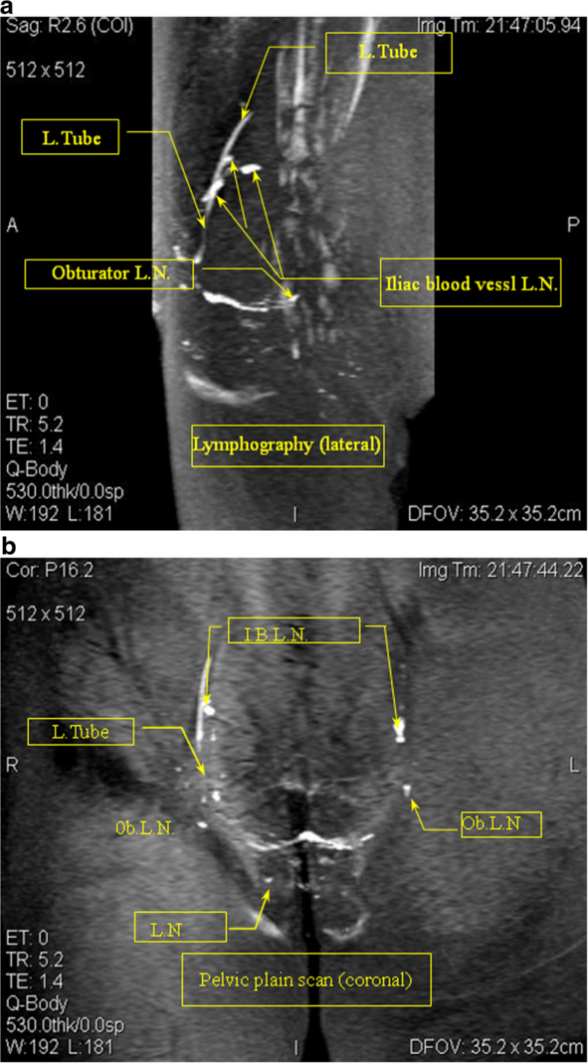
1–1: MR lymphography (sagittal view) Case 1: A 54-year-old cervical cancer patient. The MRI scan of the pelvic lymphatic system before lymphography showed no obvious lymph node metastases. The lymphography lateral map shows an irregular shape of the obturator lymph nodes (Ob. L.N.), and the iliac blood vessel lymph nodes (I.B. L.N.) show filling defects. The lymphatic shape was not continuous. MR lymphography in this patient was acquired using a slow subcutaneous injection of 15 ml Gadodiamide and 4 ml 2% lidocaine in the labia majora pudendi followed by a 30 second massage of the injection site. MRI shows the lymph nodes (L.N.) and lymphatic vessels (L.Tube). 1–2: Lymphography (coronal view) Case 1. The contrast with lymphatic (coronal view) irregular shape of the bilateral iliac blood lymph nodes (I.B.L.N.) and the right obturator lymph nodes (Ob.L.N.). A filling defect and the absence of the left inguinal lymph nodes is shown.
